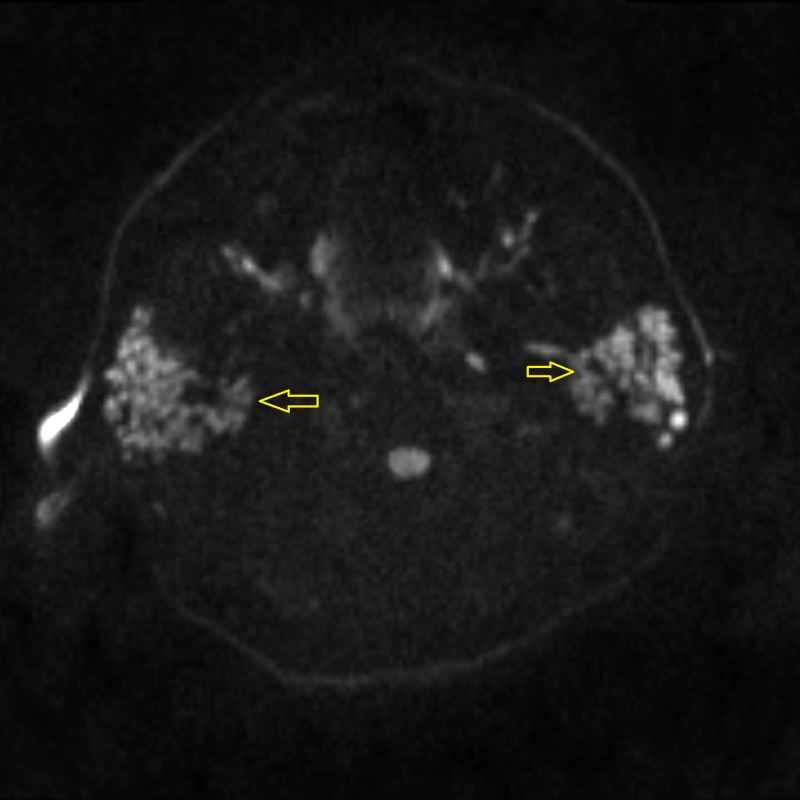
Diffusion-weighted (DW) MR imaging of the parotid gland showing hyperintense nodules in the bilateral parotid glands (arrows) suggestive of parotitis MR: magnetic resonance
